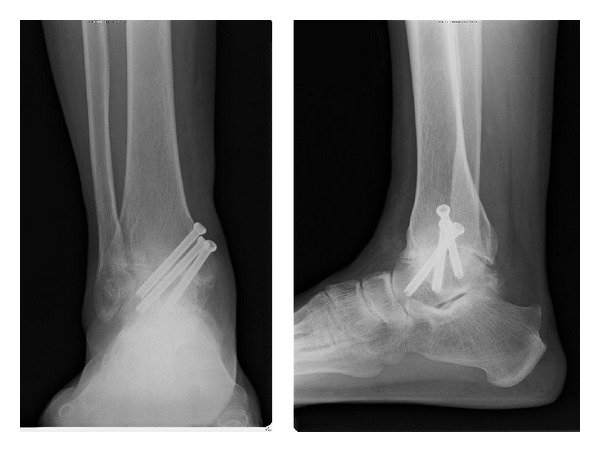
Postoperative radiographs. The bone union is adequate, and no screw loosening is observed.
